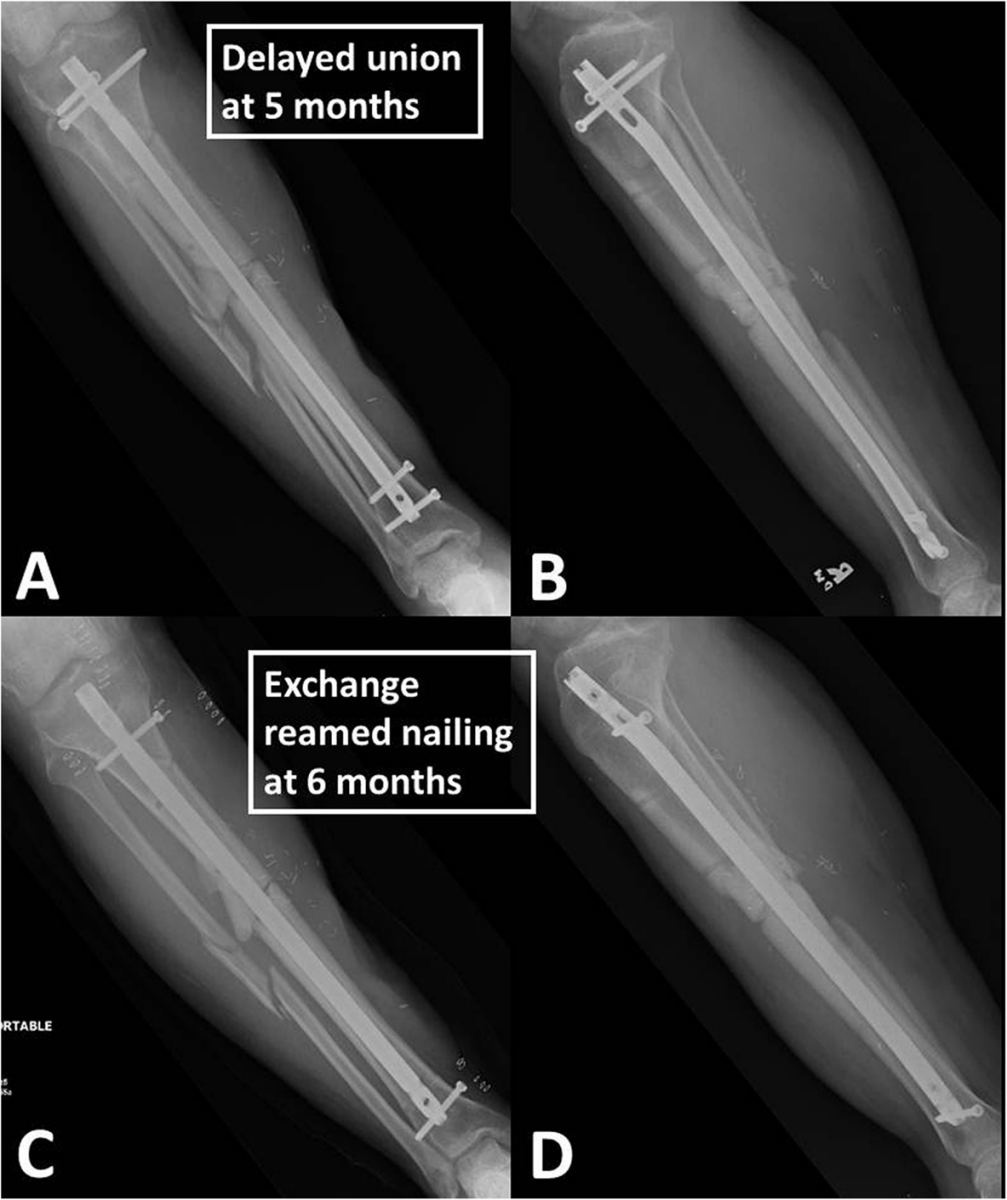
Identical case as in Figures2,3. At 5 months follow-up, the patient showed clinical and radiographic signs of a nonunion of the open tibia fracture (A, B). A surgical revision was performed by exchange reamed nailing at 6 months (C, D).
